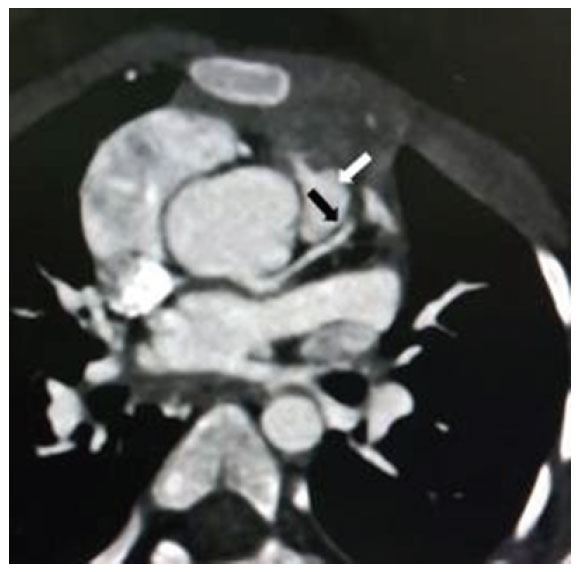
LAD passing close to pulmonic valve. Reproduced with kind permission from Prof T Ashraf:Ashraf T, Farooq F, Syed Muhammad A, Akhtar P, Khan M, Khuwaja AM, Khan MN, Karim M. Coronary Artery Anomalies in Tetralogy of Fallot Patients Undergoing CT Angiography at a Tertiary Care Hospital. Cureus 2020; 12: e10723.
